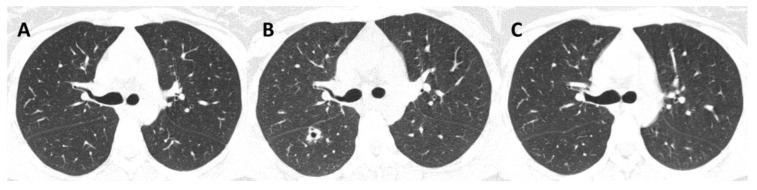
Nonenhanced chest computed tomography (CT) of a 20-year-old woman with disseminated sporotrichosis and lung lesions, at three time points (Case 14). (A) During treatment for confirmed pulmonary tuberculosis, CT shows no lung cavities. (B) Post-treatment for tuberculosis and recent diagnosis of sporotrichosis, CT shows a thick-walled cavity in the right upper lobe, with isolation of *Sporothrix* spp. from sputum. (C) Resolution of the cavity during sporotrichosis treatment.
